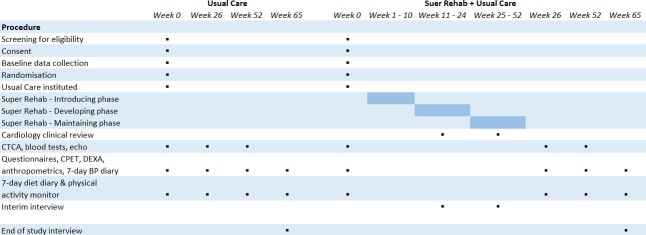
Study assessment and intervention timeline. BP, blood pressure; CPET, cardiopulmonary exercise test; CTCA, CT coronary angiohgraphy; DEXA, Dual-energy X-ray absorptiometry.
